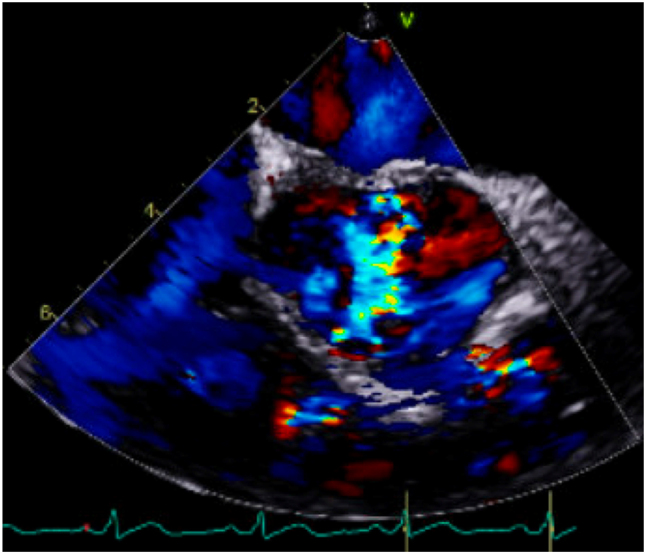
Transesophageal echocardiogram (TEE); moderate to severe aortic regurgitation.
